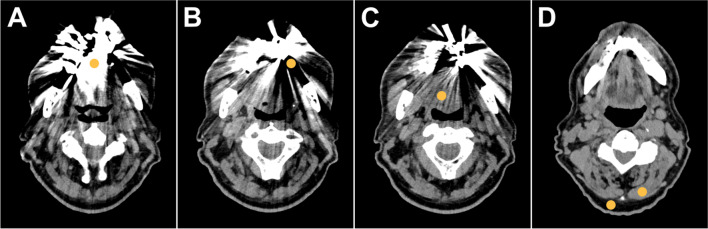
Region of interest placement in axial CT images for objective assessment of metal artifact intensity. Mean signal attenuation and standard deviation thereof were measured in the most hyperdense (A) and most hypodense artifacts (B) as well as in artifact-impaired soft tissue of the mouth floor (C). To correct for general lower image noise in high-keV reconstructions, additional measurements were performed in unimpaired muscle and subcutaneous fat tissue at the level of vertebra 2–3 (D)
